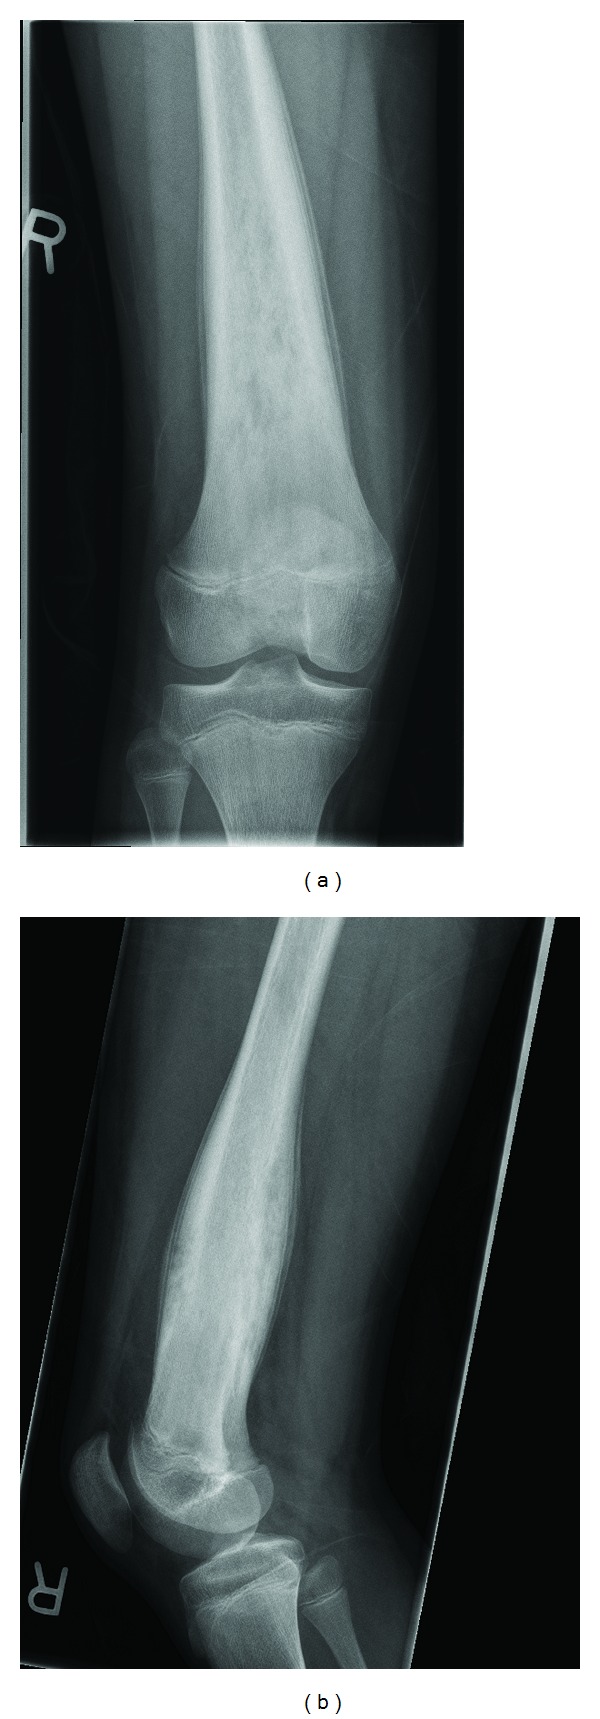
Frontal (a) and lateral (b) radiographs of the distal right femur. The lesion is shown as an expansile, fusiform mass in the distal femur, away from the growth plate. Medullary involvement is shown as patchy, moth-eaten lucencies. Smooth laminated onion-skin like periosteal reaction is also seen.
